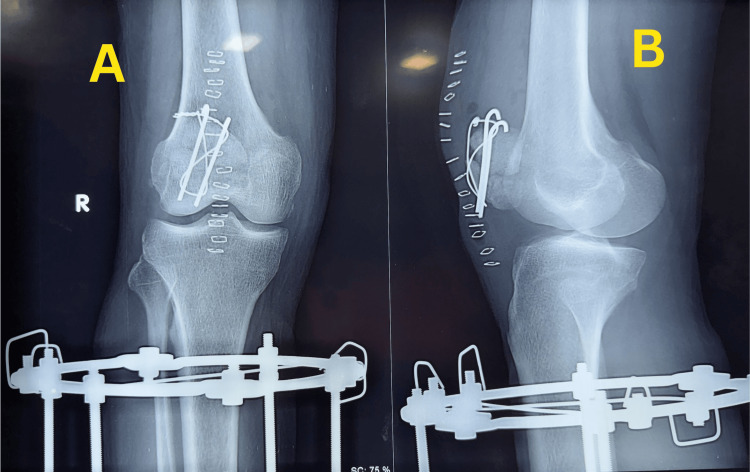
Postoperative radiographs after patellar fracture fixation Postoperative radiographs obtained on 23 December 2025, demonstrating fixation of the ipsilateral patellar fracture using modified tension band wiring following surgery performed on 21 December 2025: anteroposterior view (A) and lateral view (B).
